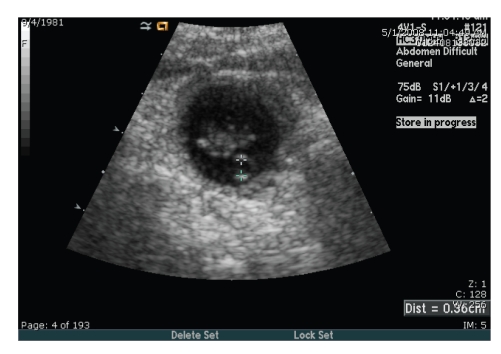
Initial ultrasound image of infrahepatic region of the abdominal wall noting a gestational sac with yolk sac and fetal pole.
